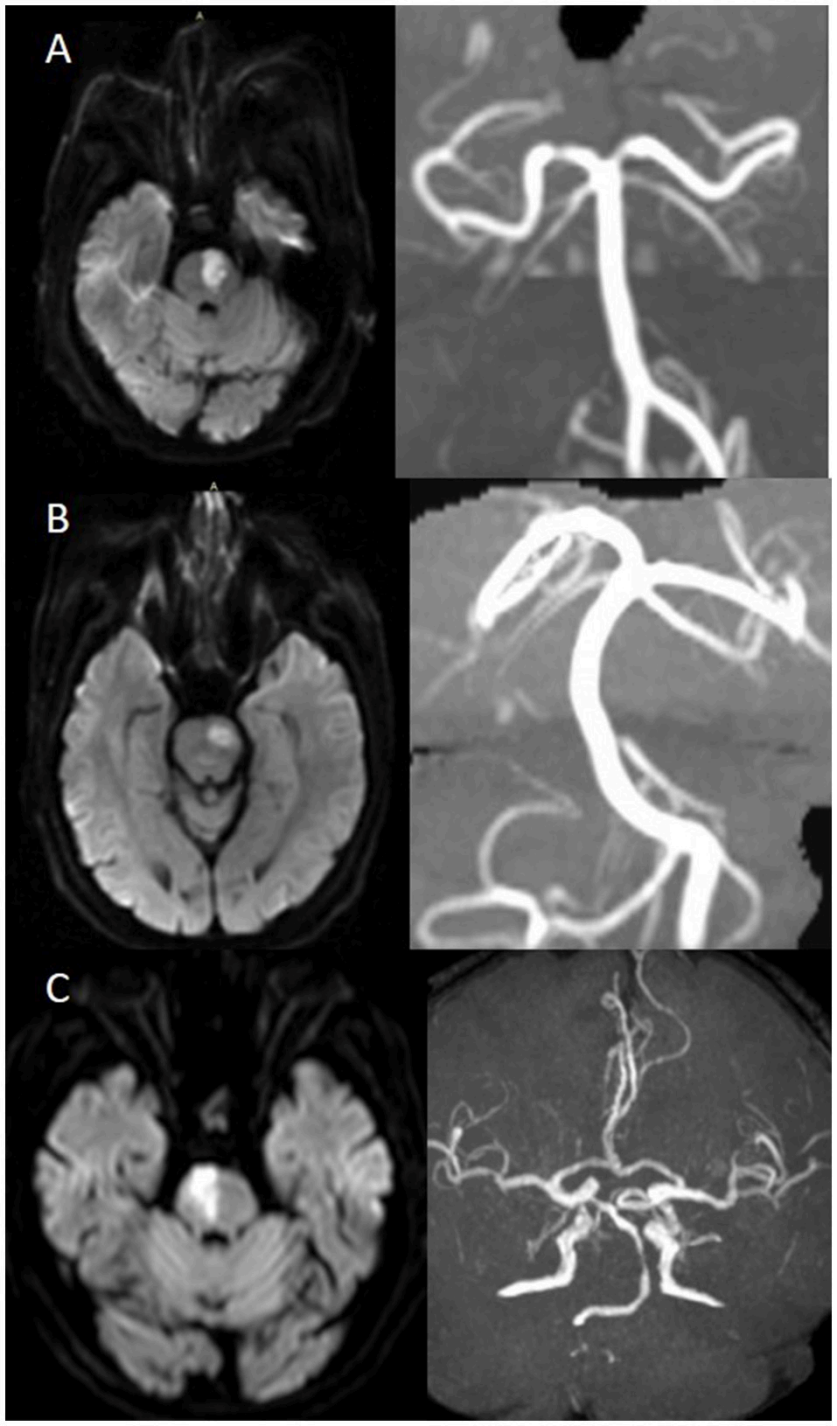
(A) BAD. DWI showing a substantial infarct that reached the pontine surface (PPI). Basilar artery stenosis was not observed on MRA. (B) SVD. DWI showing a lacunar pontine infarct (LPI) not reached the pontine surface, basilar artery stenosis was not observed on MRA. (C) LAOD. DWI showing PPI. MRA showing severe stenosis of the basilar artery.
